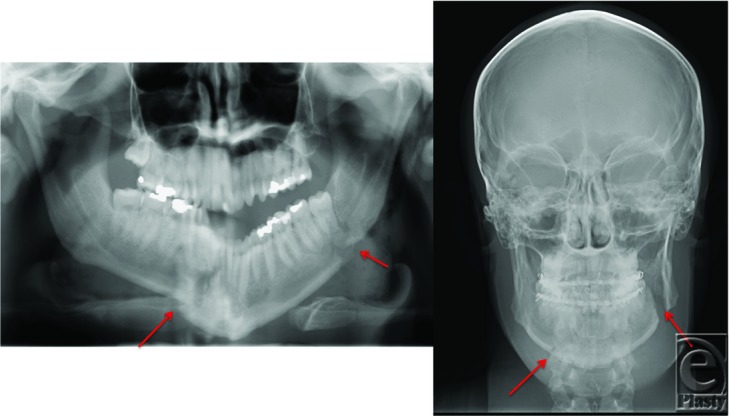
Panoramic (*left*) and anterior-posterior (*right*) radiographs demonstrating displaced right mandibular parasymphysis/body fracture and left mandibular angle fracture (*arrows*).
